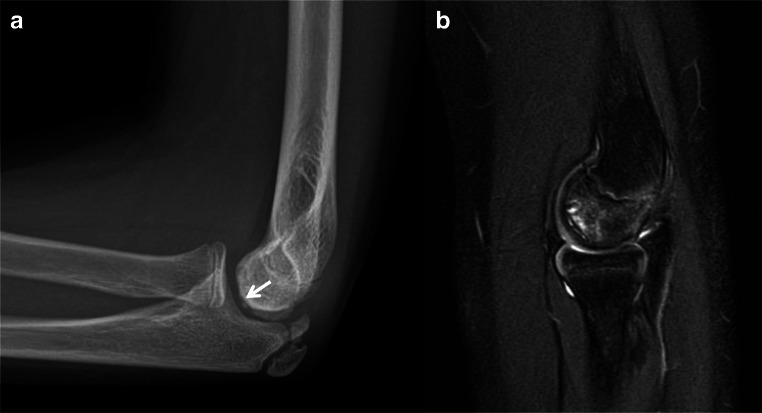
a Lateral radiograph of the elbow demonstrates osseous irregularity and sclerosis of the capitellum with subtle fragmentation (arrow). b Sagittal T2 MR image more clearly demonstrates collapse of the capitellar subchondral bone plate with subjacent cyst-like changes consistent with an osteochondral lesion
